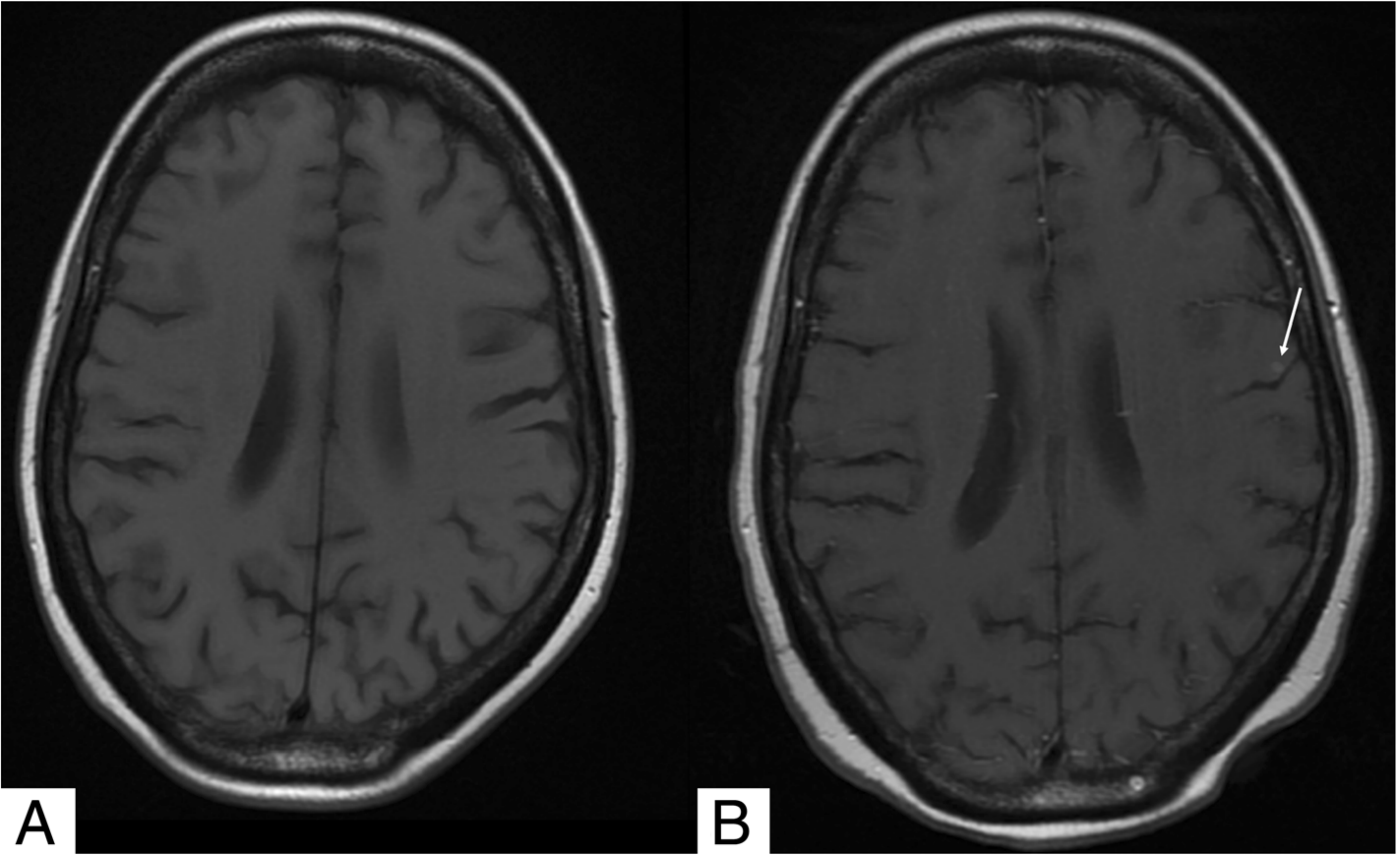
Axial T1 magnetic resonance image of the brain. a Precontrast. b Postcontrast. A small white focus is seen on the motor strip within the left hemisphere after contrast is added (*white arrow*). The same area is unremarkable before contrast. This contrast-enhancing lesion is a probable tuberculoma. The location of this lesion corresponds to the patient’s right-sided epilepsia partialis continua seizures
